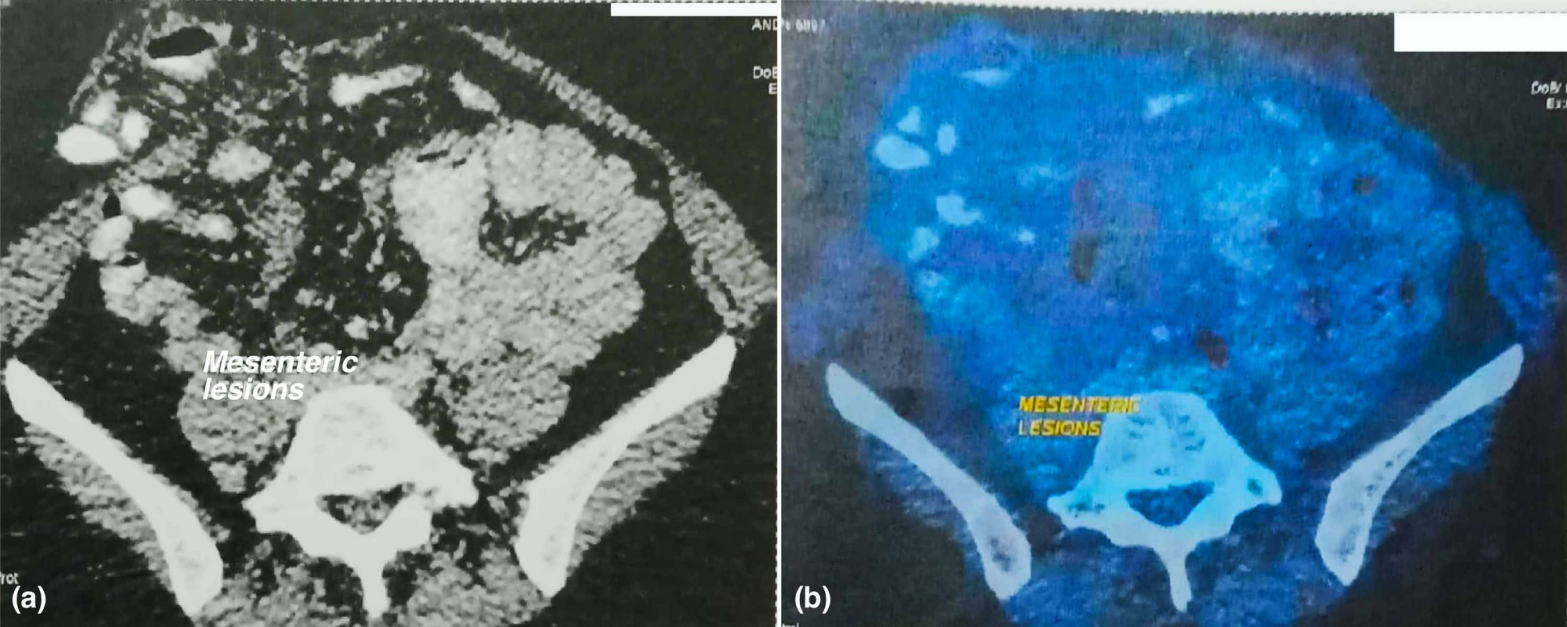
(a) Contrast-enhanced CT abdomen image shows mesenteric lesions with peritoneal fat stranding. (b) Corresponding fused PET-CT image shows patchy areas of soft tissue thickening in the mesentery adjacent to the ileostomy site with mild increased metabolic activity, measuring ~ 28 x 18 mm (standardized uptake value [SUV] Max = 1.5). PET-CT, positron emission tomography-computed tomography.
